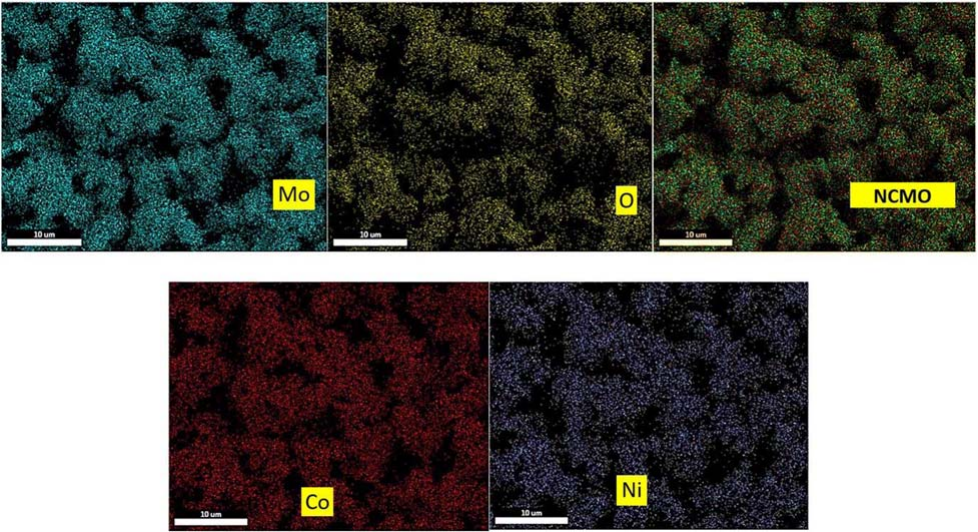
X-ray mapping analysis of Spinel-nanostructured NCMO.
